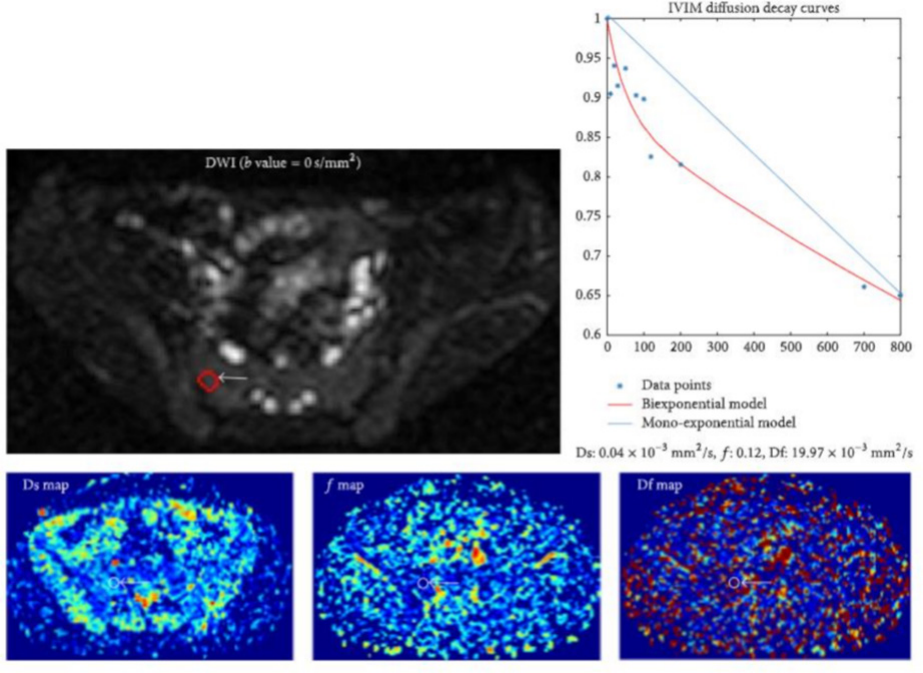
IVIM DWI in a patient with axSpA (figure reproduced from Zhao et al^
